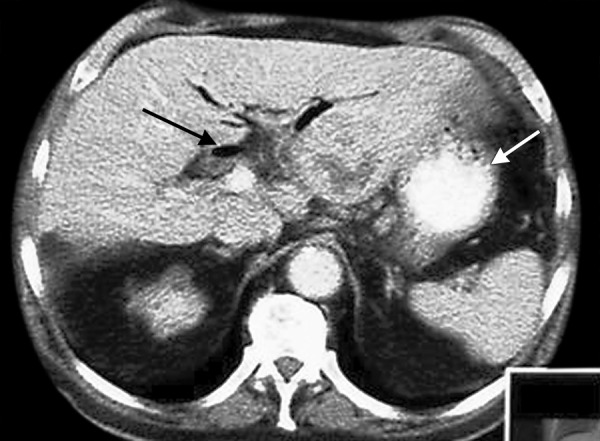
Abdominal contrast-enhanced computed tomography. In the left hepatic lobe (segment II), a heterogeneous, hypodense area is visible (white arrow), 50 × 55 × 35 mm, with tiny, partly connected liquid spaces. The dilated extrahepatic biliary ducts are indicated (black arrow). There is visible air inside the intrahepatic biliary ducts, CBD and gallbladder
